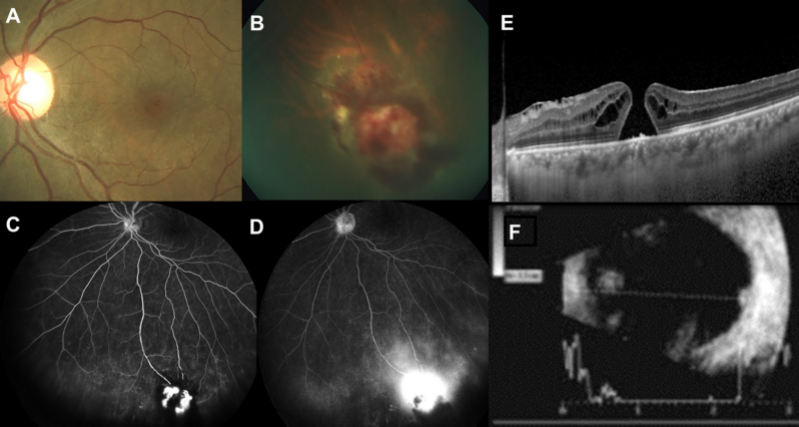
A) Color fundus photo of the left eye shows a small full-thickness macular hole. B) The inferotemporal quadrant shows a retinal vasoproliferative tumor, seen as a pinkish red mass with retinal hemorrhages. C, D) The fundus fluorescein angiography in the early phase showed hyperfluorescence corresponding to the tumor with a few areas of blocked fluorescence due to hemorrhages with progressive leakage at the late phase. E) Spectral domain optical coherence tomography of the left eye shows a small full-thickness macular hole with cystic spaces at the edges of the hole. F) Ultrasound revealed an acoustically solid tumor with medium to high internal reflectivity with tumor dimensions of 3.8 mm (longitudinal basal diameter) x 2.6 mm (vertical basal diameter) x 1.6 mm (apical thickness).
